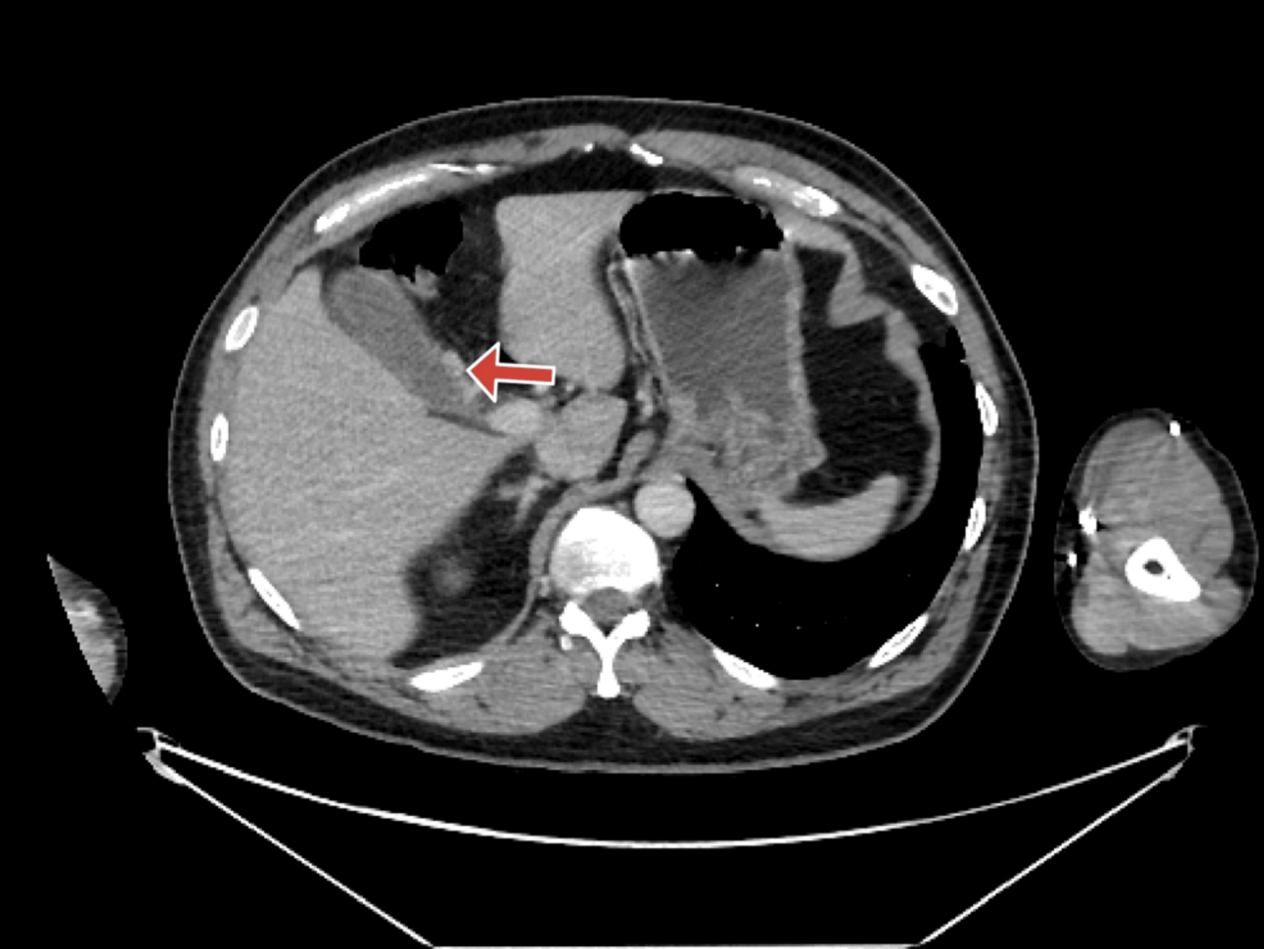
Computed tomography (CT) image of the cystic artery Contrast-enhanced abdominal CT, axial view in the portal venous phase, showing the cystic artery (arrow).
